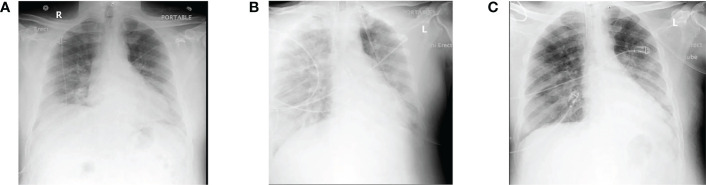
(A) Representation of the initial X-ray when the patient was admitted; (B) at day 6 of hospitalization representing severe and unremitting hypoxia and fever, with continued bilateral lung infiltration; (C) lung X-ray of cytokine filtration therapy at the first day demonstrating continuous infiltration with increased aeration with alleviated right lower lobe consolidation. Reproduced with permission from (80).
